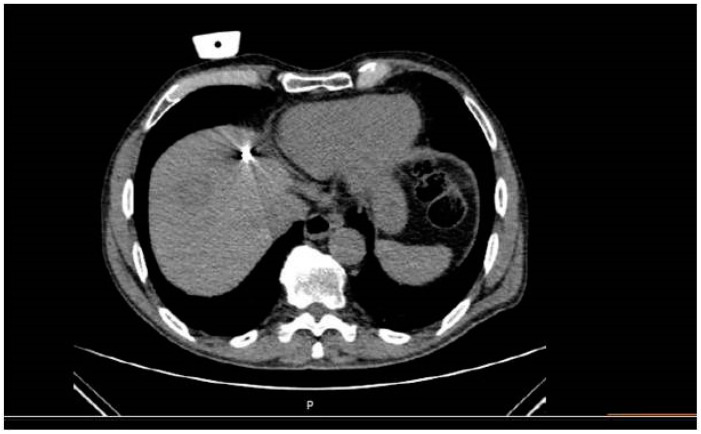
Same patient as in Figure 1 and Figure 2: Computed tomography axial scan during evaluation of the placement of the antenna in the target lesion and potential gas bubbles dispersion.
